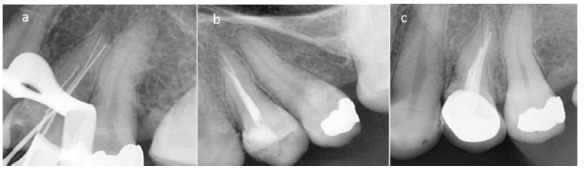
Periapical radiograph of the tooth with the restoration at the 12-month follow-up
